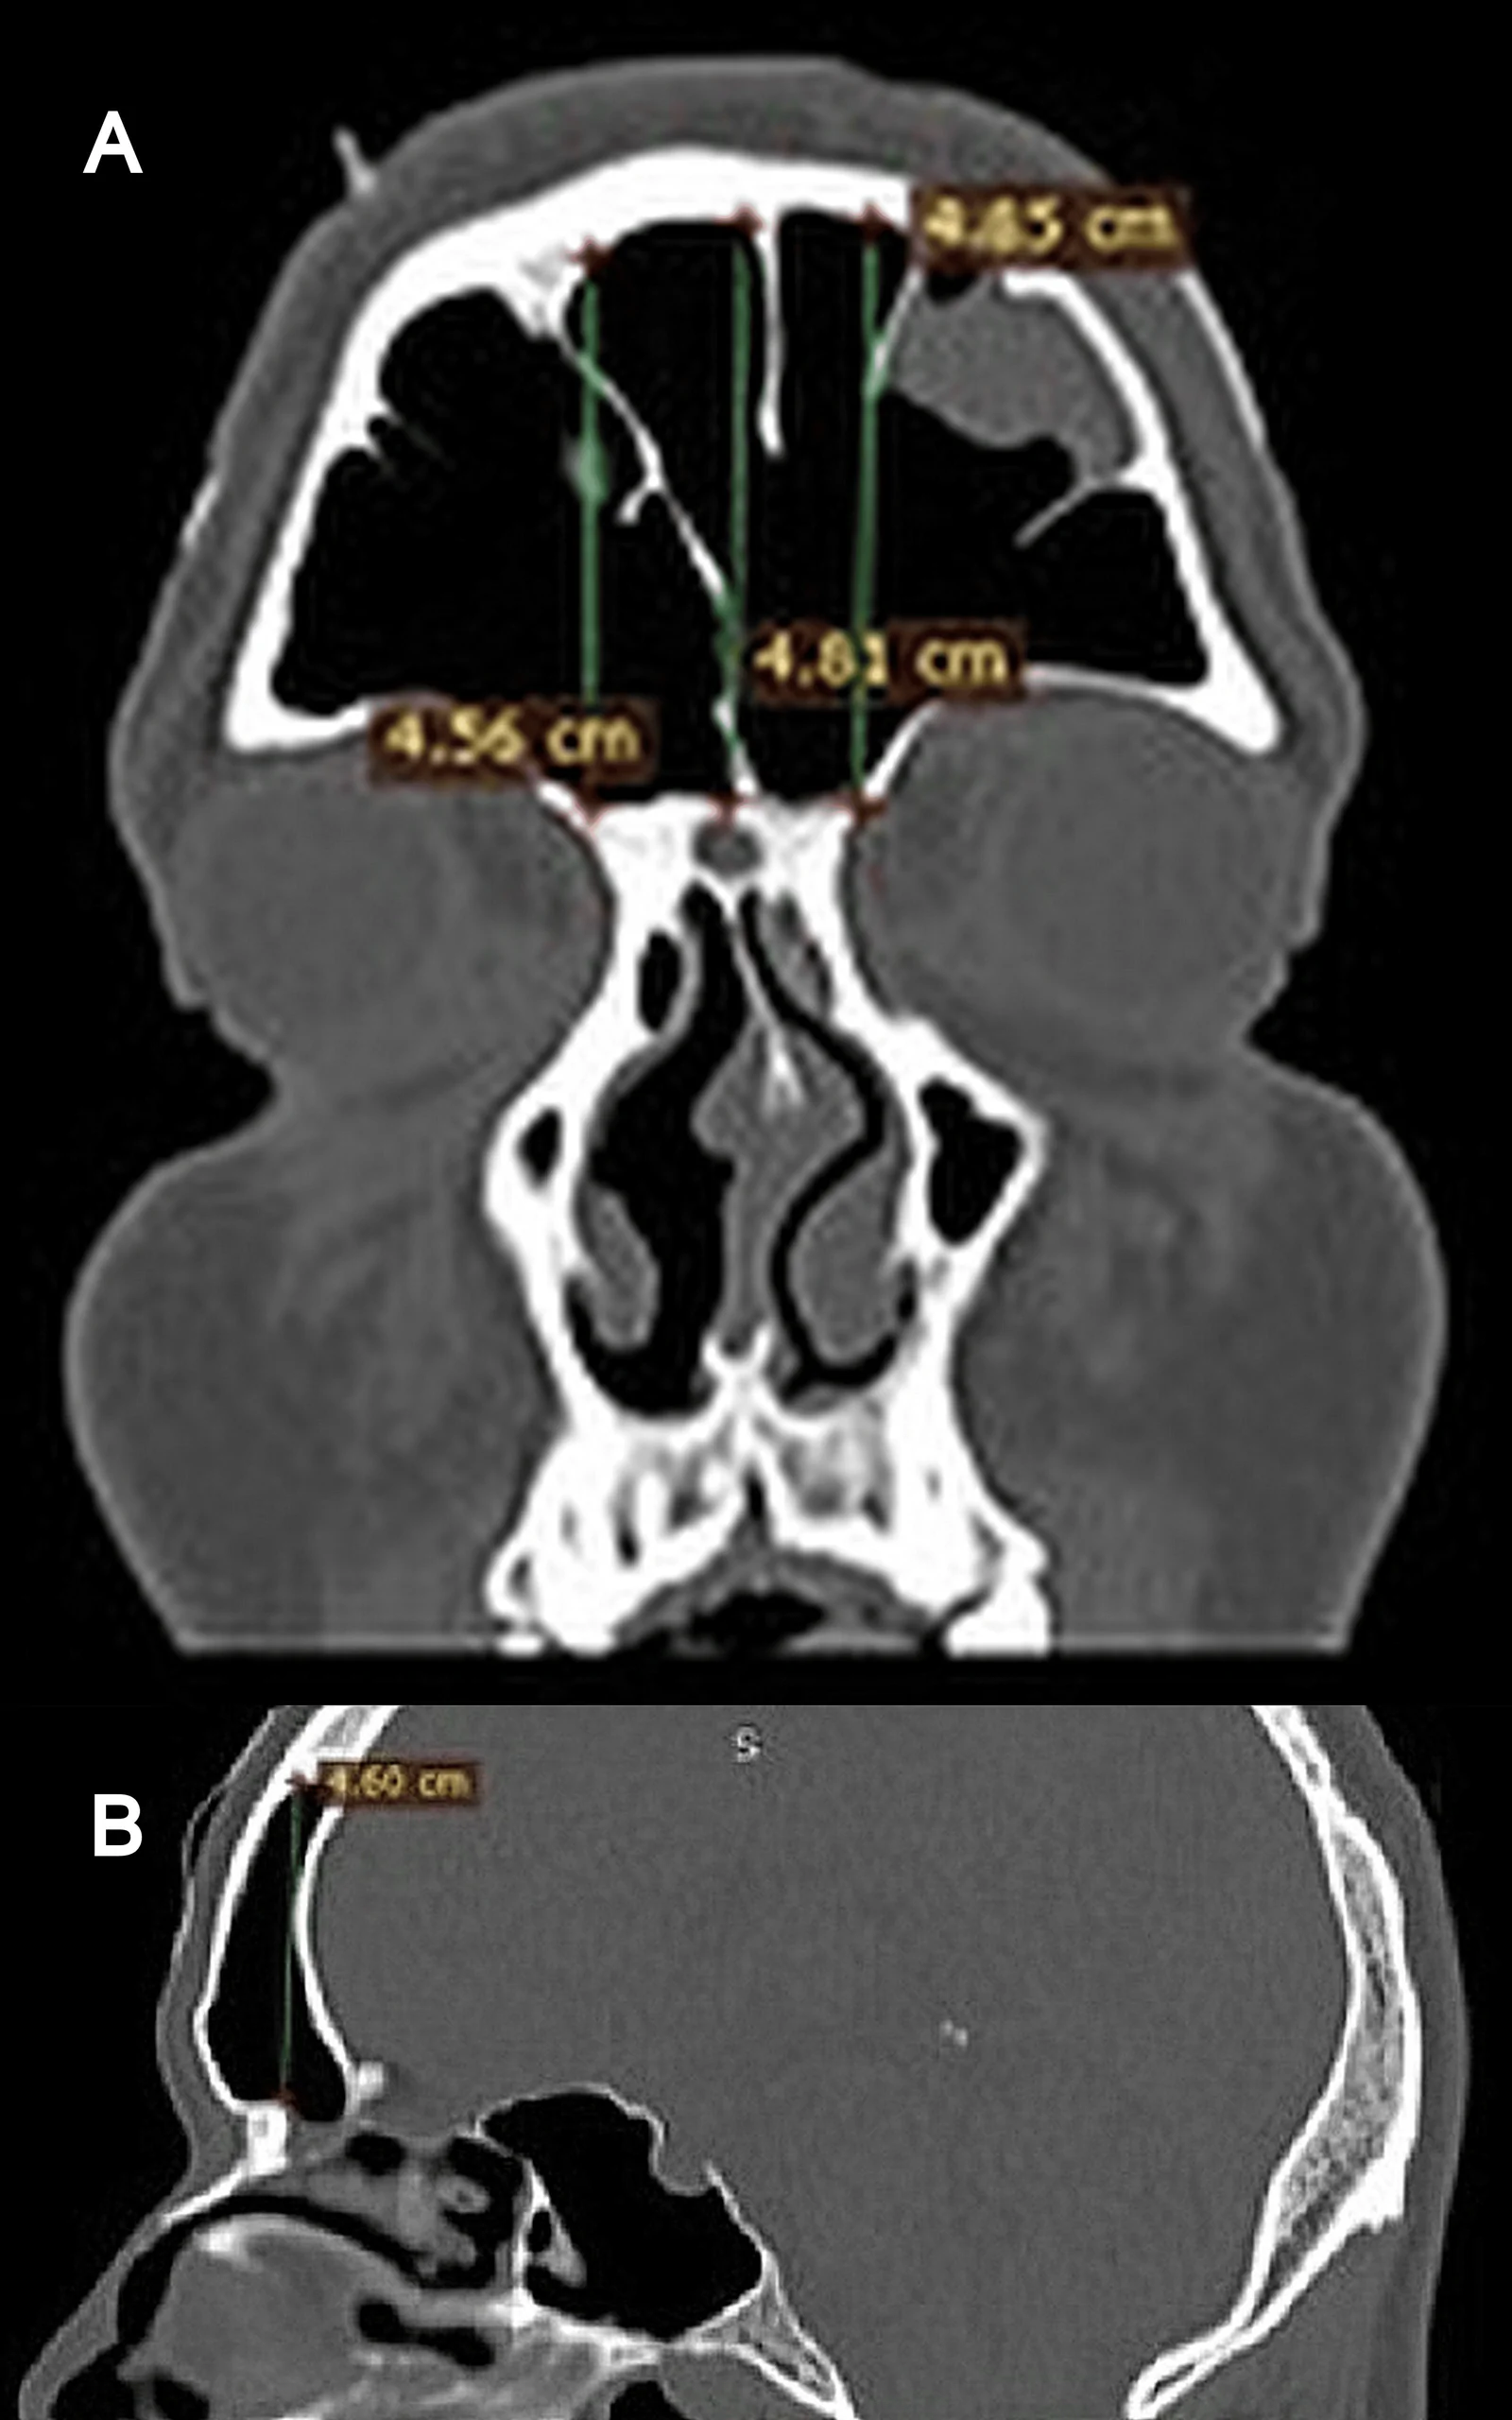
Non-contrast CT scan of nose and paranasal sinuses depicting neumosinus dilatans (A) Coronal view showing average craniocaudal dimension of 4.74 cm and (B) Sagittal view showing craniocaudal dimension of  4.60 cm of the frontal sinus
